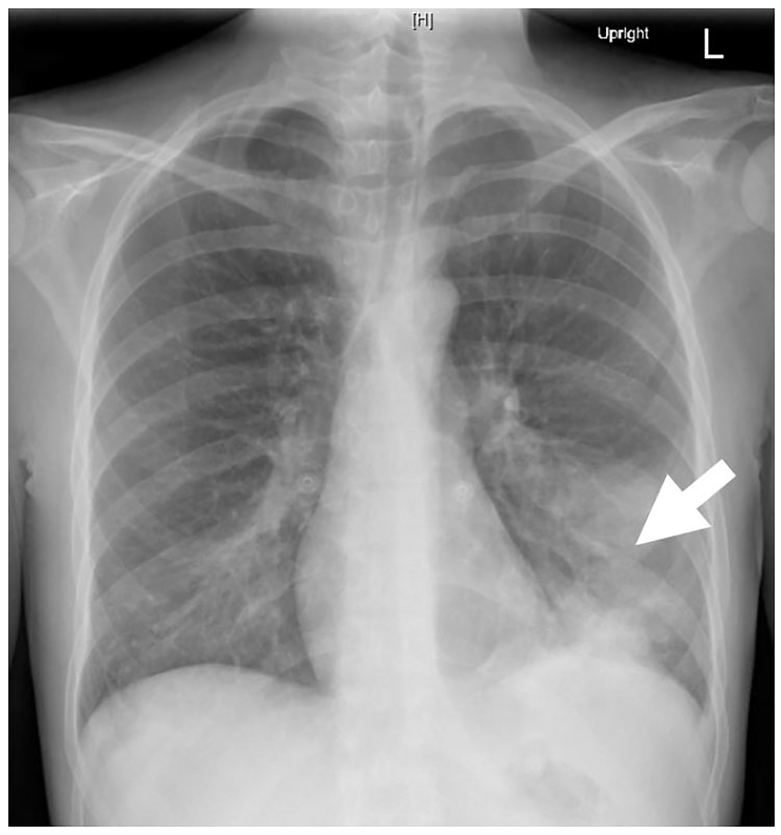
This plain film of the chest demonstrates a left lower lobe consolidation (arrow).
